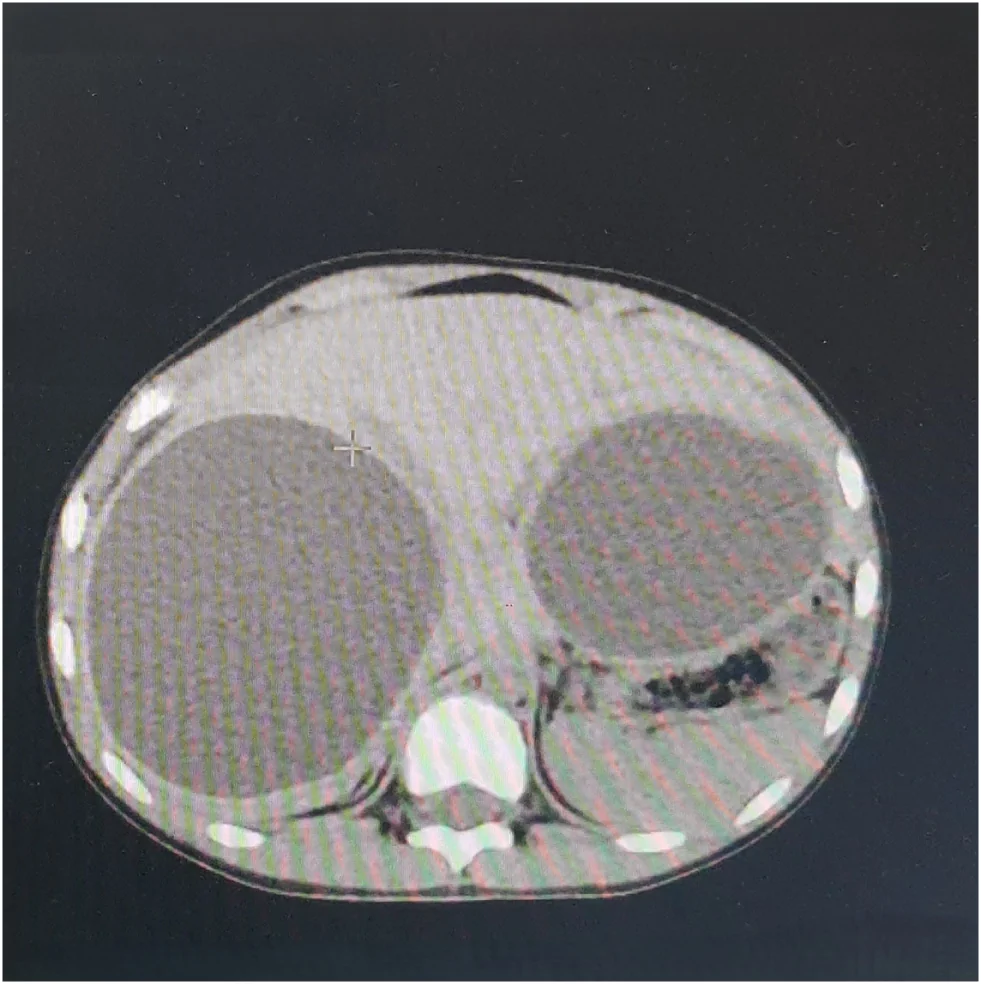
Axial computed tomography (CT) scan of the abdomen demonstrating multiple large, well-defined, unilocular cystic lesions in the hepatic parenchyma, characteristic of giant hepatic hydatid cysts. The cysts exhibit homogeneous fluid density without internal septations, causing significant displacement of surrounding structures.
